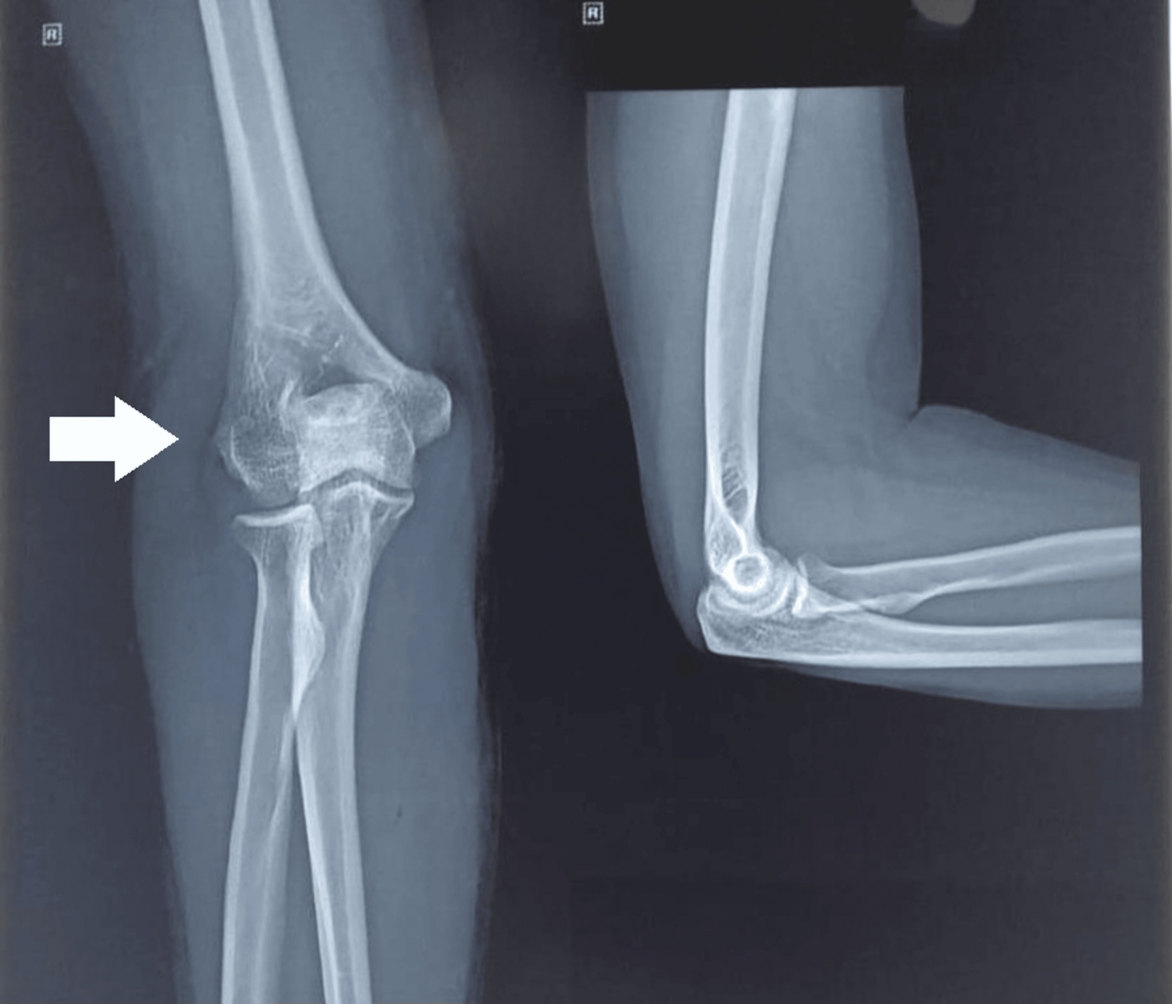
Elbow Radiograph Osteophytes, calcific tendinopathy, or arthritic changes not visualised
